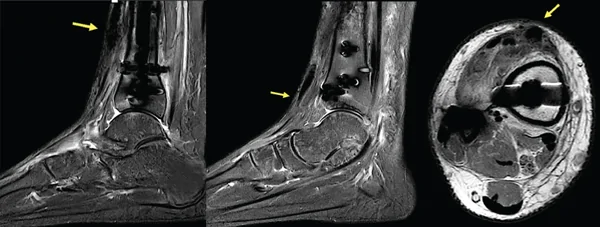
MRI demonstrating postoperative continuity of the anterior tibial tendon.
